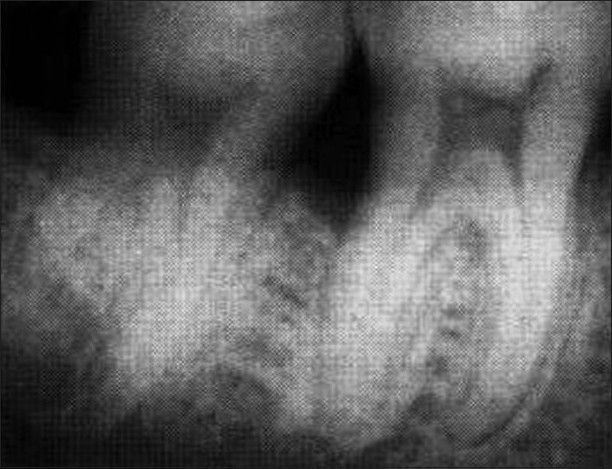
Radiograph of experimental site at 6 months
